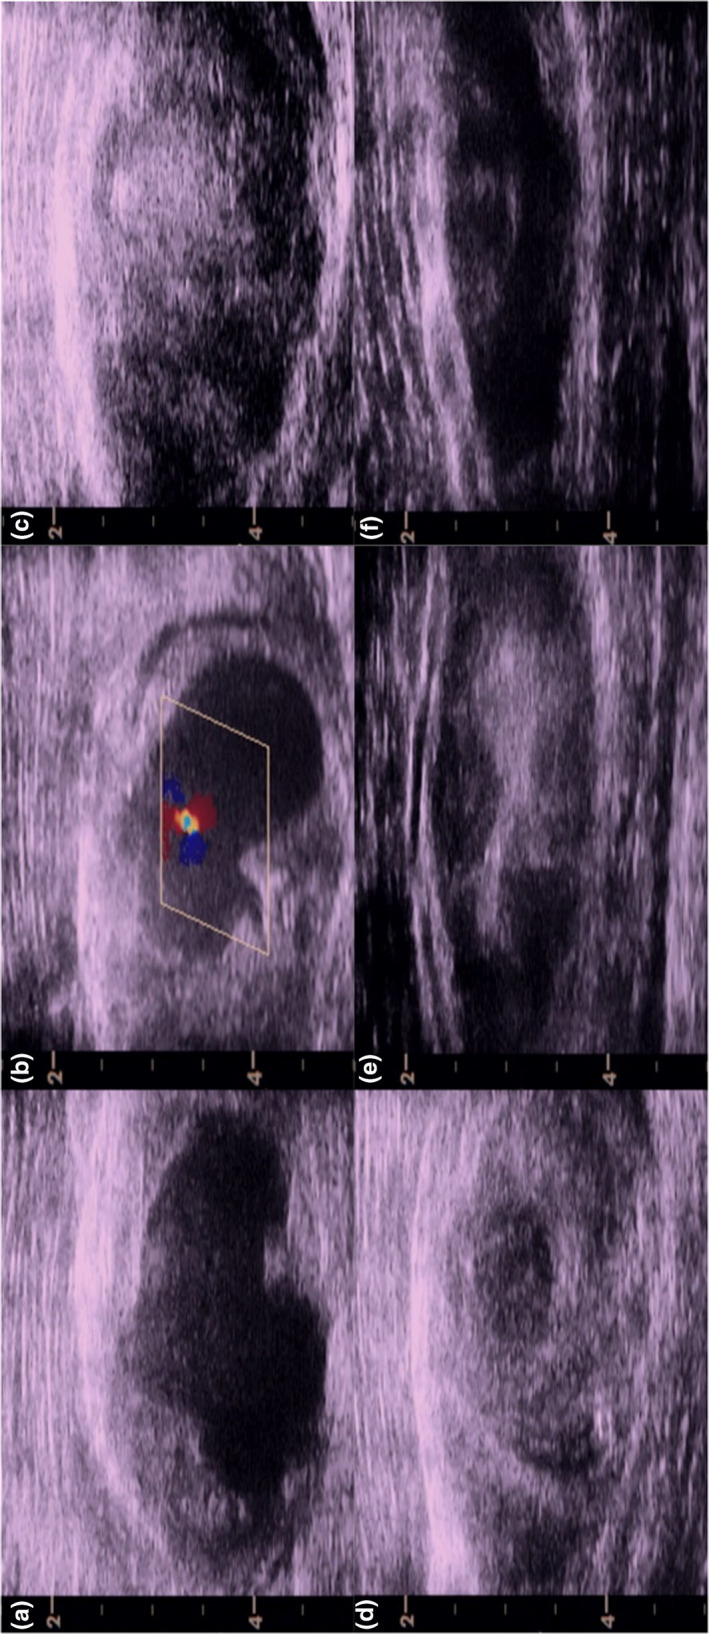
Transverse ultrasound images of right vastus lateralis showing the intramuscular hematoma five (a), six (b), eight (c), nine (d), twelve (e), and nineteen (f) days after percutaneous microbiopsy. Color in (b) reflects arterial flow in the heterogeneous mass. Y‐scale is measured in cm
